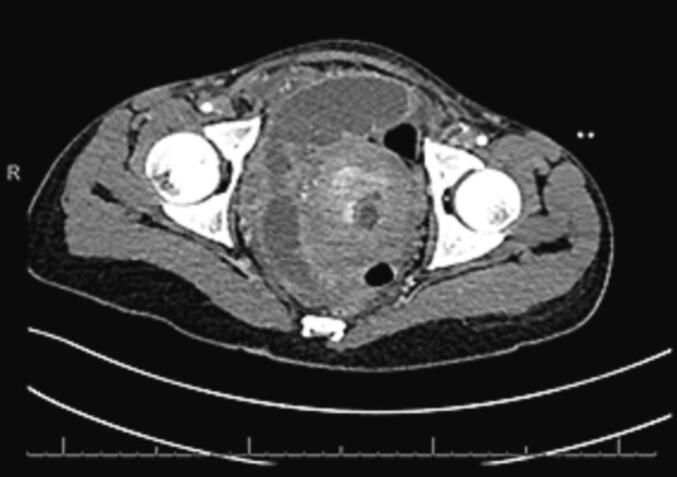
Contrast enhanced CT abdomen showing dilated loops of proximal small bowel with air-fluid levels and pneumatosis intestinalis.
